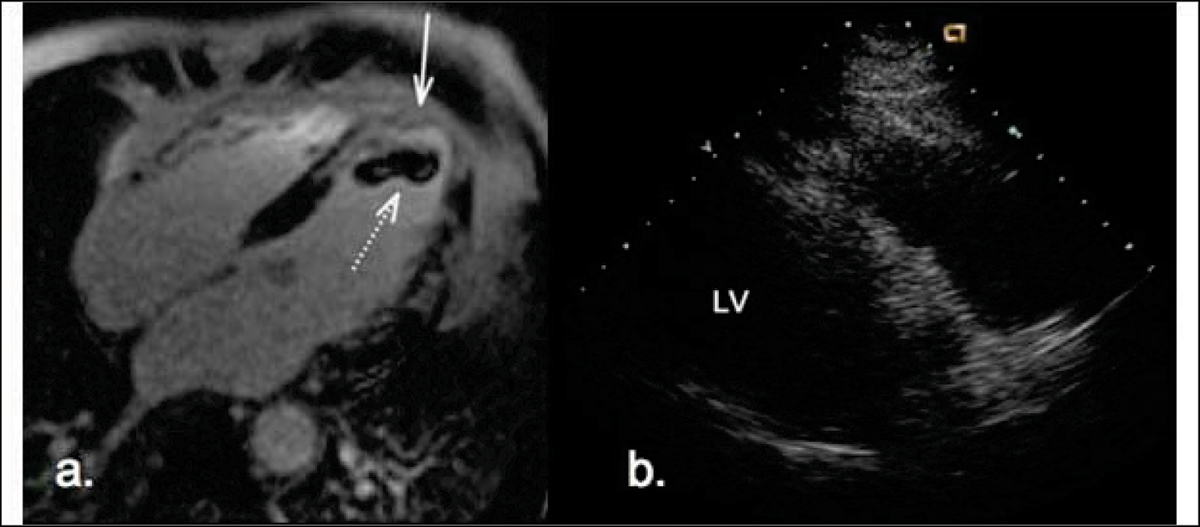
a. On PSIR Turbo FLASH there is a thrombus (dashed arrow) within the apical region of a mildly dilated left ventricle. This is located adjacent to a transmural infarct (arrow) b. No thrombus is identified on echocardiography.
